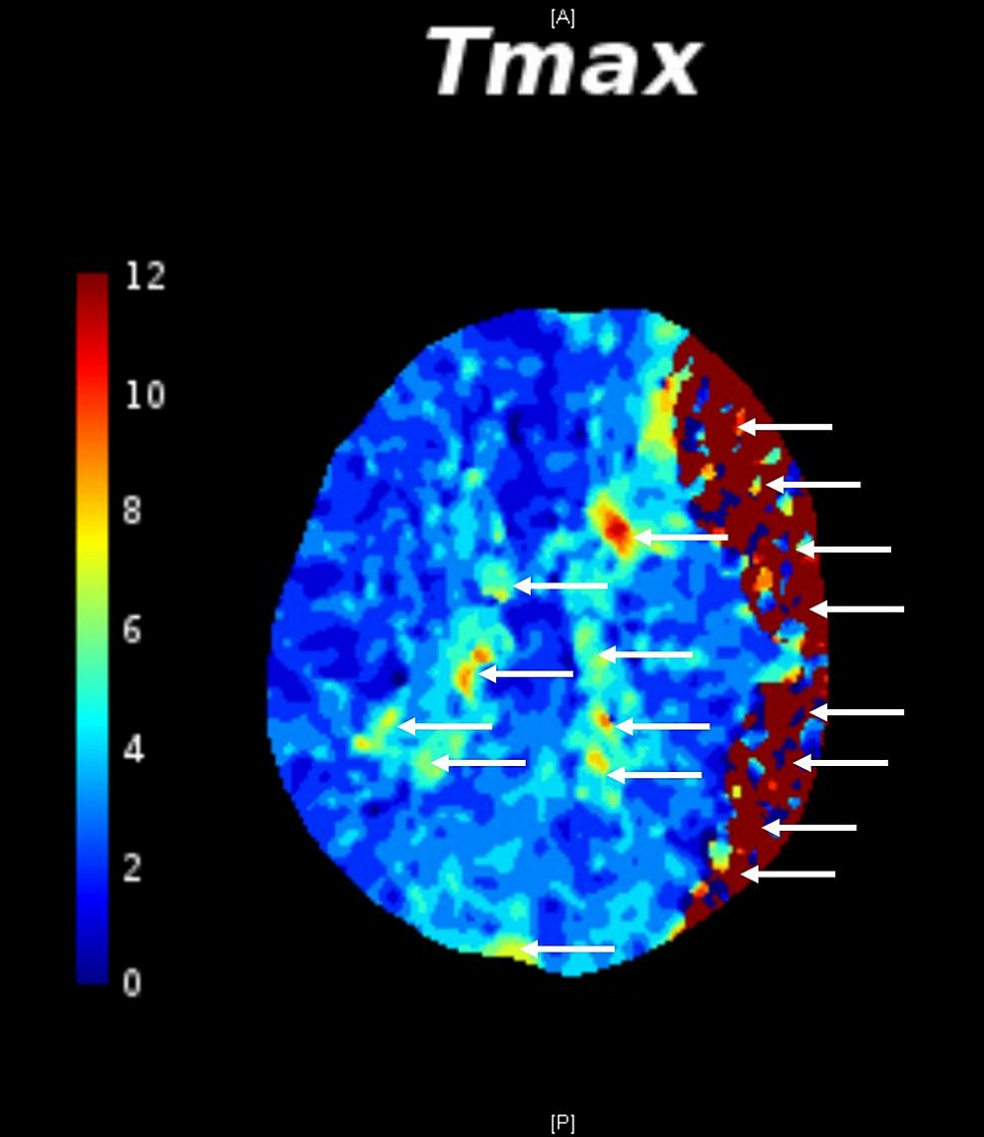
CT cerebral blood flow study confirms subdural hematoma and reveals areas of ischemia/penumbra that are salvageable. CT: Computed tomography.
